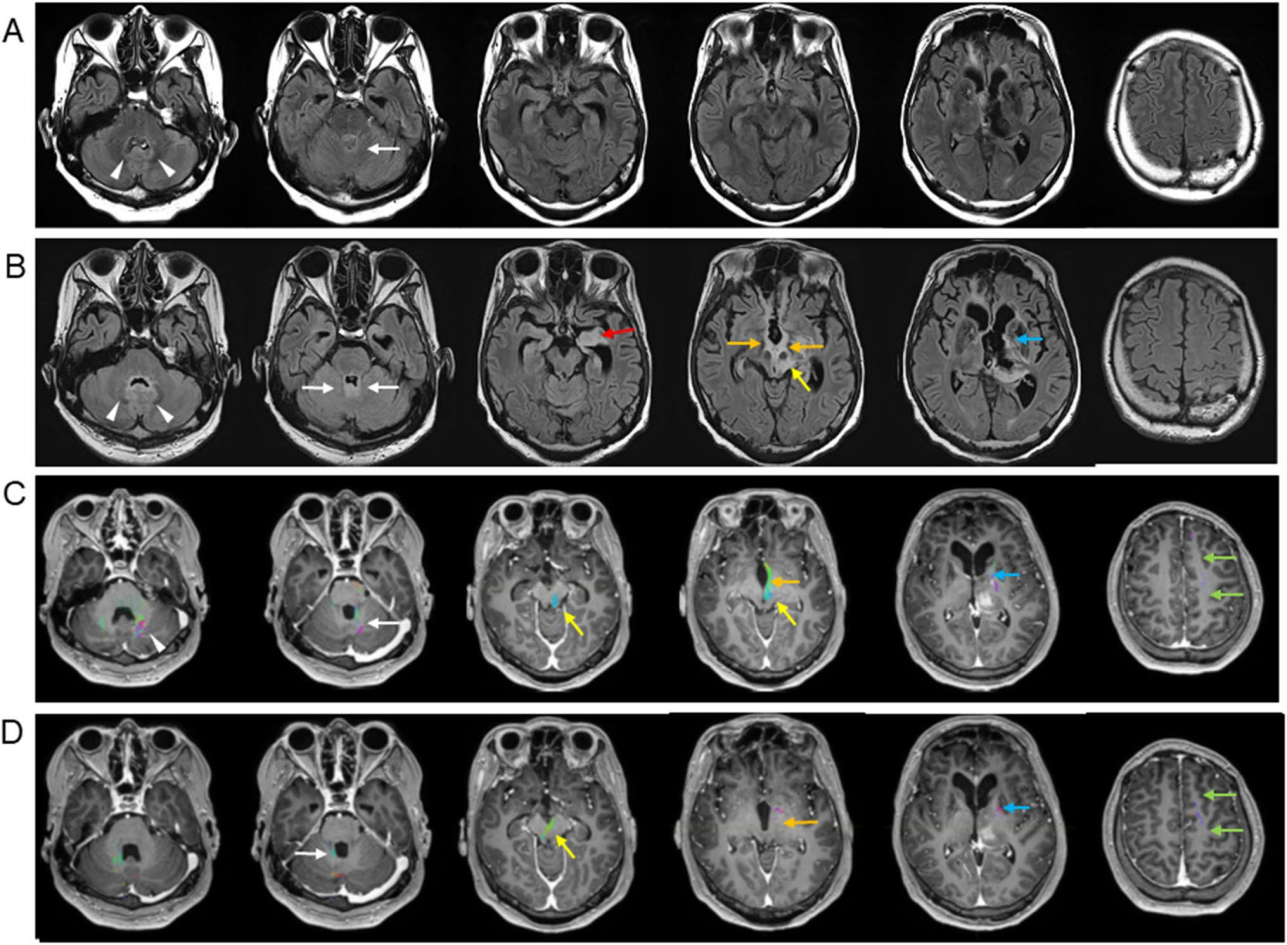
The pattern of further progression after recurrence in the DN on FLAIR images in case 8 with left thalamic glioblastoma, *IDH*-wild type. FLAIR images at the time of the appearance of abnormal signals (A) and at delayed diagnosis after 3 months (B). For delayed diagnosis, the lesion at the hilus of the DN (arrowhead) and SCP enlarged (white arrow). Recurrence at the primary site and abnormal signals in the tectum and tegmentum, including the red nucleus (RN) (yellow arrow), internal capsule (blue arrow), mammillary body (orange arrows), and amygdala (red arrow), were noted. Preoperative fiber tracking fused with the Gd-T1WI from the left DN (C) shows that the tract from the DN (arrowhead) runs through the SCP (white arrow), tectum, and tegmentum, the RN (yellow arrows) and internal capsule (blue arrow), and ends in the frontal lobe (green arrows). Alternatively, preoperative fiber tracking, fused with Gd-T1WI from the right DN to the left frontal cortex (D), was run through the SCP (white arrow), tectum, and crossed the tegmentum. It connected the left RN (yellow arrow) and internal capsule (blue arrow) and ended in the frontal lobe (green arrows). Tractography from a single VOI set in the right DN (C) and that from seed VOI to target VOI were demonstrated in C and D, respectively
